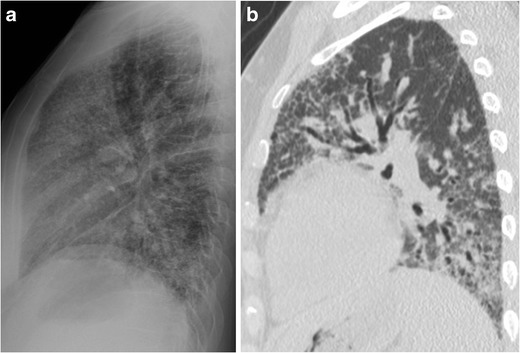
53-year-old woman with chronic hypersensitivity pneumonitis. The lateral chest radiograph (a) projects fibrosis as well through the retrosternal clear space as at the lung bases. A corresponding sagittal CT slice (b) is also shown
